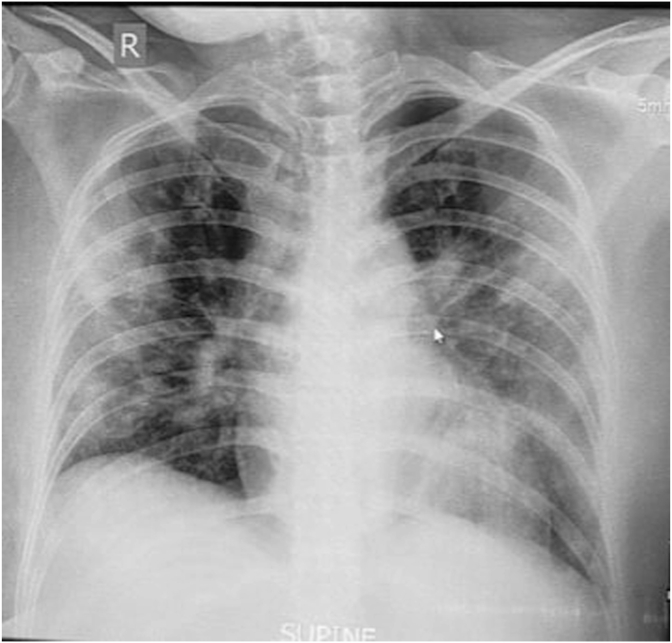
Plain chest X-ray showed the appearance of GGO in the periphery of both the lung and multifocal consolidation parahilar and paracardial in the right lung.
